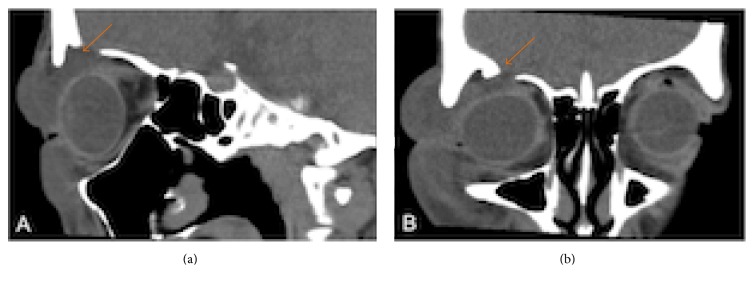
Orbital CT scans ((a) sagittal scan, (b) coronal scan) revealed an area of bone discontinuity in the right orbital roof with adjacent cephalocele (arrow).
